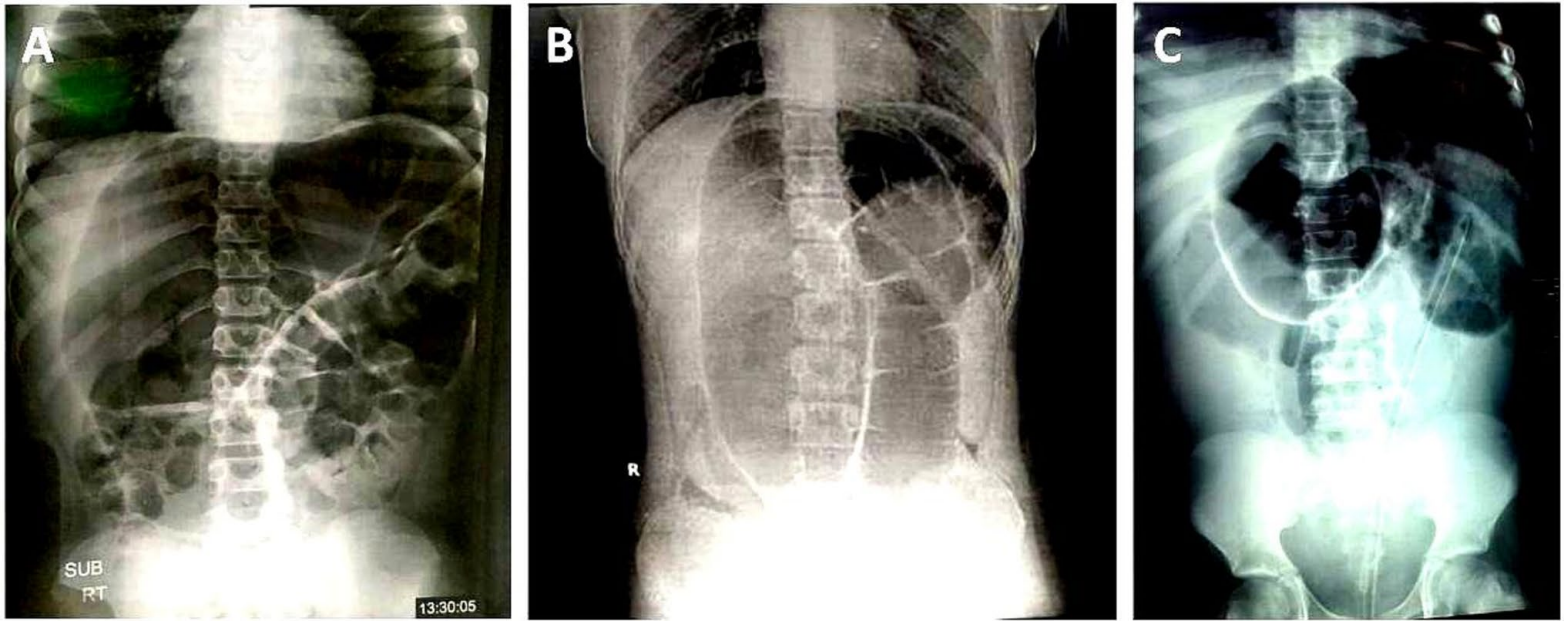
Three different supine abdominal X-rays showing Coffee Bean Sign (massively dilated and gas-filled sigmoid colon which formed inverted U-shaped shadow) and Liver Overlap Sign (the distended sigmoid extends and projects over the liver shadow). A) A case of Hirschsprung disease, B) A case of chronic constipation, C) A case of chronic constipation with rectal tube insertion
